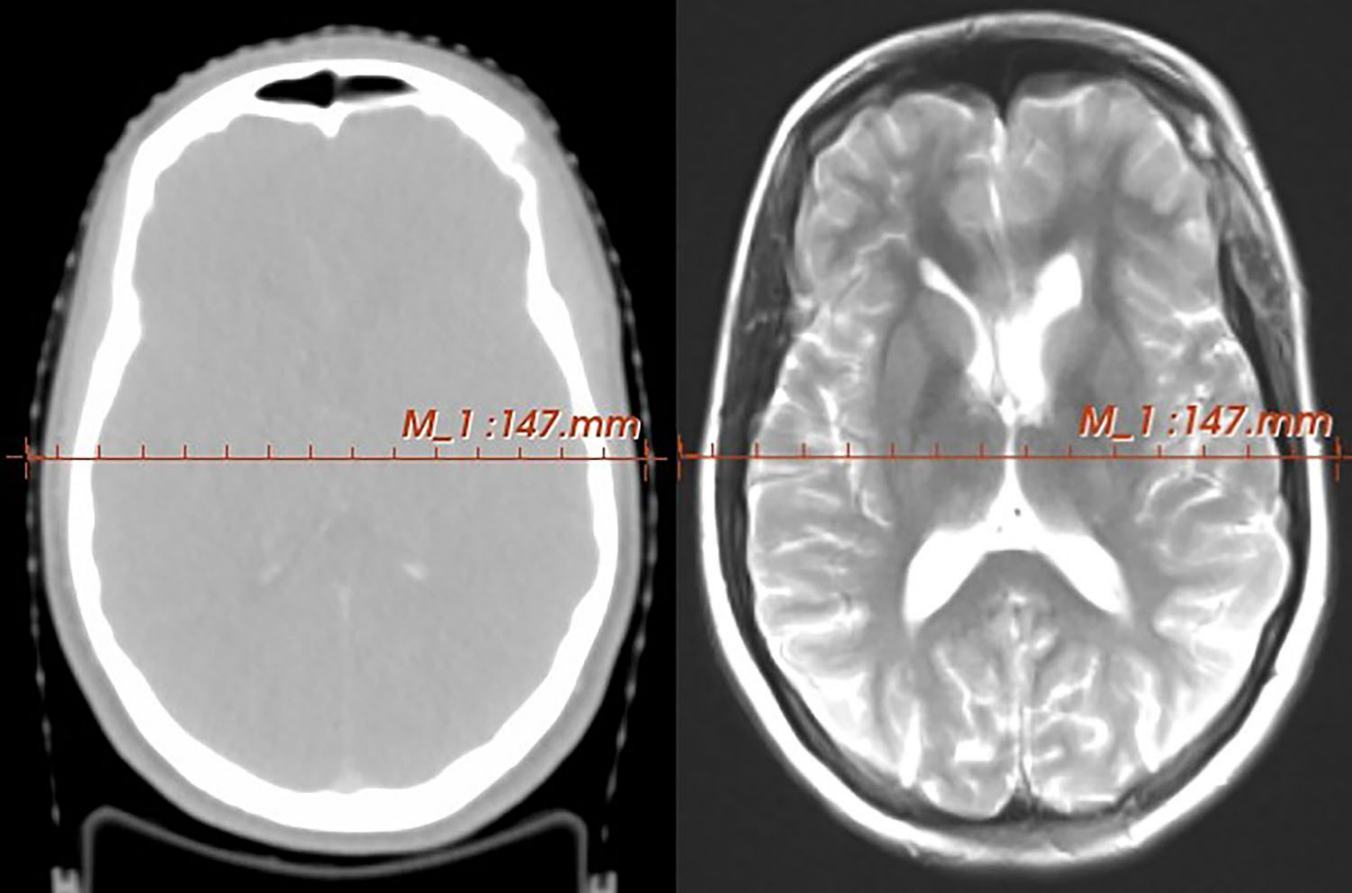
Considered lines for pixel values evaluation in CT and MR images.
